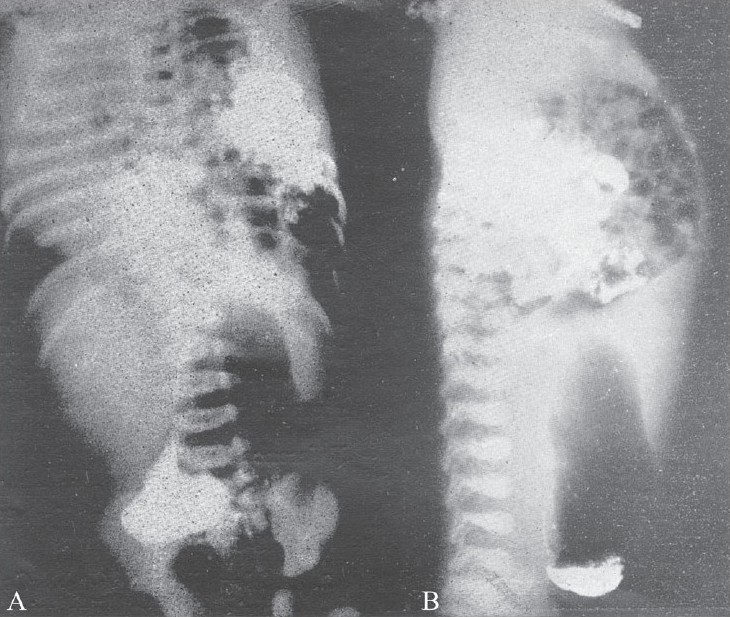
Diaphragmatic hernia. AP (A) and lateral (B) radiographs show shift of the heart and mediastinum with Lipiodol-outlined bowel
